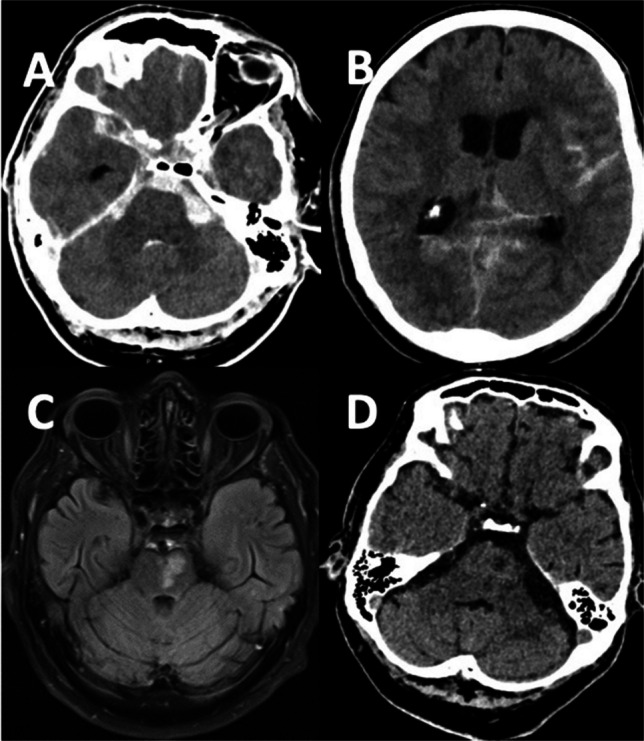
Case 2, 67-year-old man. Computed tomography (CT) with axial views (A, B), performed immediately after the onset of symptoms including severe headache nausea, vomiting, and loss of consciousness, revealed a subarachnoid hemorrhage, Fisher grade 3, with a large prepontine blood clot, more prominent on the left side than on the right side, accompanied by acute hydrocephalus, without evidence of an aneurysm on CT-angiography or digital subtraction angiography performed on the same day (not shown here). Axial FLAIR MRI sequences obtained after the event revealed a small left-sided infarction on the ponto- mesencephalic border (C), likely due to the occlusion of a small basilar artery perforator supplying the brainstemCase 3 (Fig. 4): A 56-year-old woman presented with sudden onset of severe bifrontal headache, neck pain, sweating, and nausea. A cranial CT scan revealed an extensive SAH, Fisher grade 3. CT-angiography did not show any cerebral aneurysm. Upon arrival, the patient was intubated and ventilated due to a deterioration in her neurological status. Transfemoral pangiography on the same day did not reveal an aneurysm or another bleeding source. An emergency EVD was placed via a right frontal burr-hole to treat hydrocephalus, and the patient was admitted to the neurocritical care unit. She was extubated the next day, and no neurological deficits were observed. Daily transcranial Doppler ultrasounds showed no evidence of vasospasm. An MRI performed 10 days after the event revealed a small infarction in the left posterior putamen, likely caused by the occlusion of a small perforator artery from the lateral lenticulostriate branches of the middle cerebral artery on the left side. Clinically, the patient remained without neurological deficits. A follow-up angiography performed two weeks after the initial presentation did not identify any bleeding source. The EVD was successfully removed without the need for the placement of a ventriculoperitoneal shunt. She remained neurologically stable throughout her stay. By the time of discharge, 20 days after the admission, the patient was awake, fully oriented, in good general condition, and her neurological examination was unremarkable.
